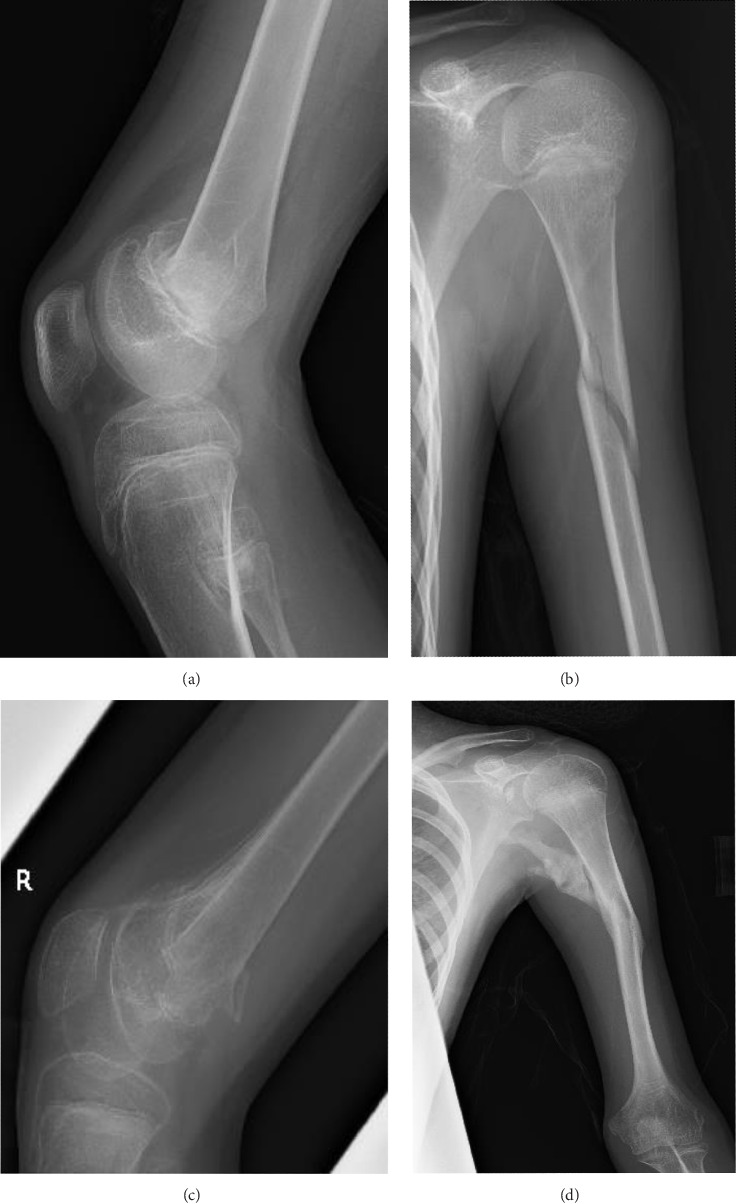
Bone fractures in association with generalized seizures (a) A radiograph of the right knee made at the time of injury showing a displaced Salter–Harris type II physeal fracture of the distal femur. (b) A radiograph of the left humerus made at the time of injury showing a mid-shaft spiral fracture of the humerus. (c) A radiograph of the right knee made 6 months after the injury showing sagittal rotational malunion of the distal femur without apparent HO. (d) A radiograph of the left humerus made 6 months after the injury showing the proper alignment and well union of the fracture. Note abundant heterotopic bones connecting the mid-shaft humerus and the glenoid neck of the scapula.
